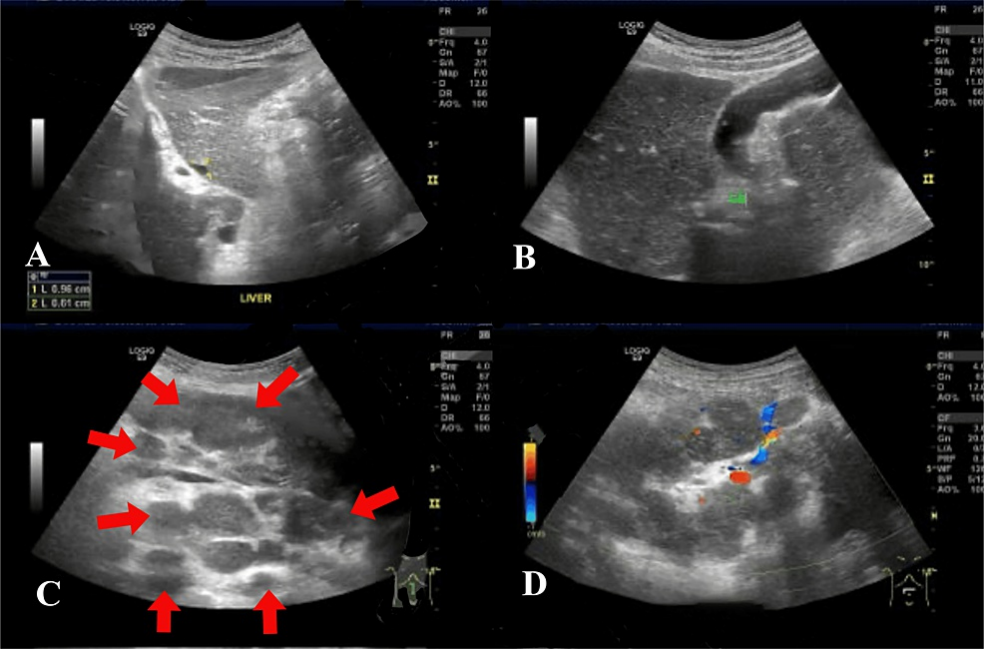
Abdominal ultrasound FIGURE 1A and 1B and 1C and 1D: Abdominal ultrasound revealed a hypoechoic mass in the abdominal cavity extending from below the pancreas to the level of the umbilicus, measuring approximately 17.3×15.3×8.7cm, with an irregular shape and unclear boundaries, appearing to be composed of multiple hypoechoic areas fused together.
